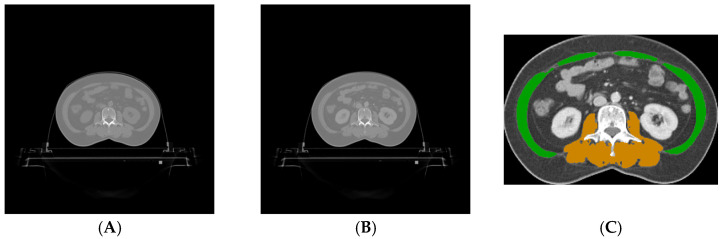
Both the plain (A) and contrast-enhanced (B) CT images at the third lumbar vertebral level of a case in the test set. The gray scales of the CT images are the same. The corresponding gold standard on the contrast-enhanced CT image (C) includes both the abdominal muscles (green) and the paraspinal muscles (orange). For better visualization, the annotated image was cropped, and the gray scale was adjusted.
